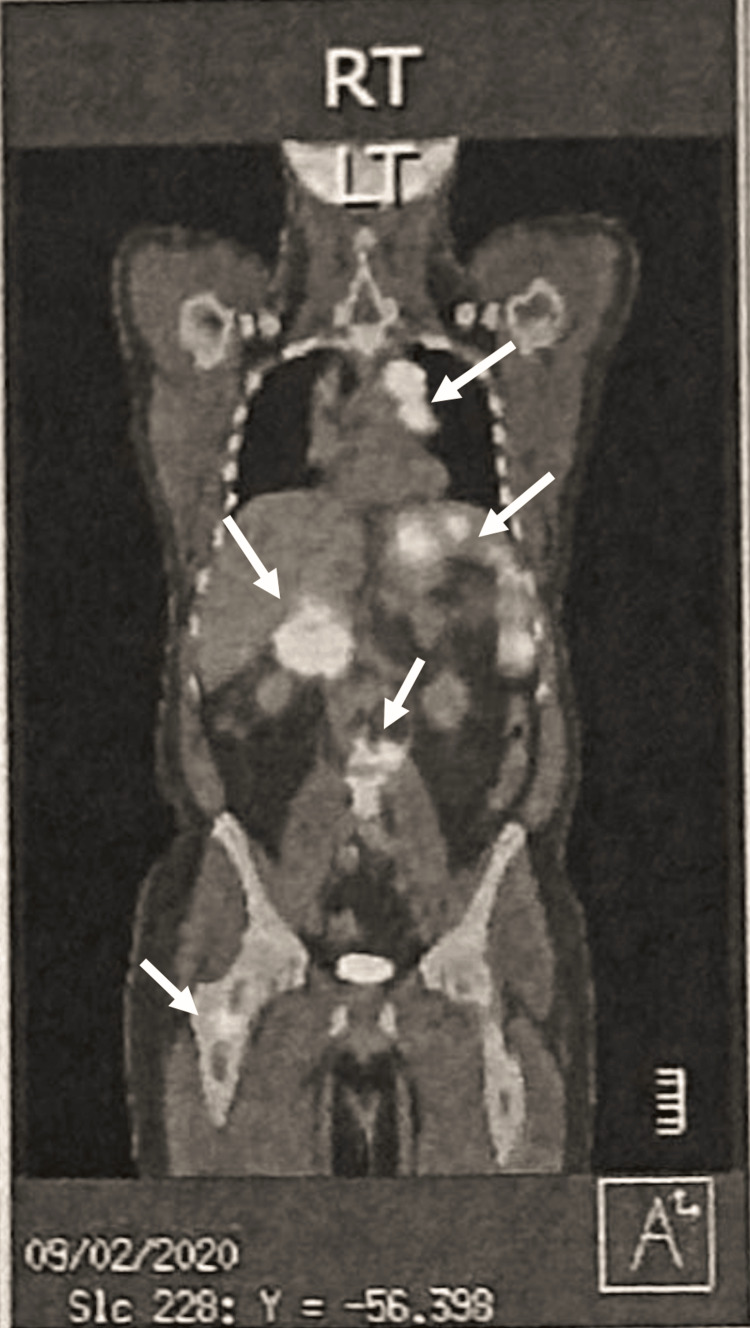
PET-CT Skull to Thigh (Month 3) Right supraclavicular lymph node with an SUV of 4.1; right subclavicular lymphadenopathy with an SUV of 3.2; substantial perivascular mediastinal mass with SUV of 35.4; mediastinal lymphadenopathy with with an SUV of 1.2; hypermetabolic right paratracheal lymph node with an SUV of 4.4; multifocal hypermetabolic splenic masses with an SUV of 19.8; hypermetabolic upper abdominal lymphadenopathy in the portacaval and mesenteric root distribution with an SUV of 28.1; right intertrochanteric femur lesion with an SUV of 7.5.
